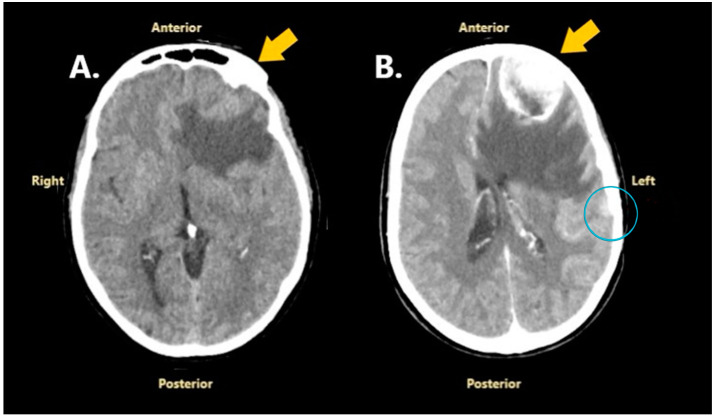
A cranial CT scan performed in May 2024: (A)—without contrast enhancement; (B)—with contrast enhancement. The yellow arrows point to the left frontopolar meningioma, and the blue circle surrounds the left frontoparietal recurrent lesion.
